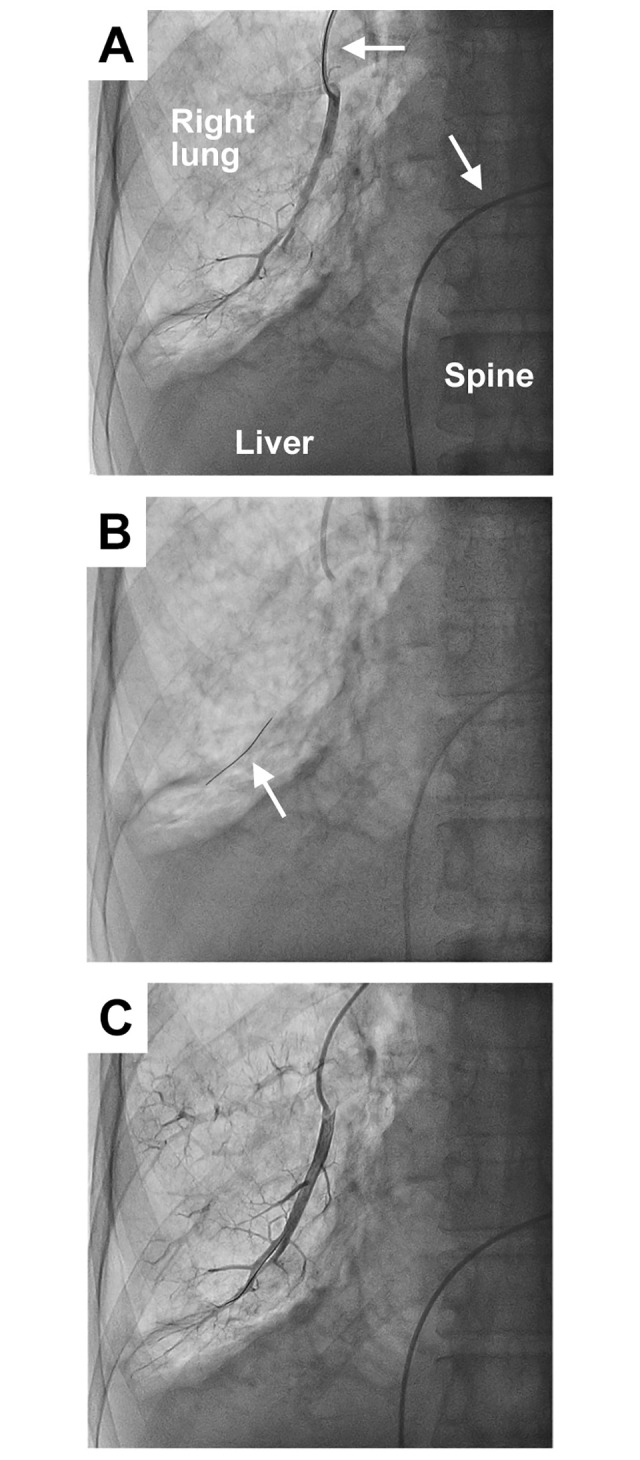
Representative X-ray frontal images during cardiac catheterization. (A) The pulmonary arteries in the lower lobe of the right lung are shown using contrast medium. The suitable pulmonary artery to facilitate the placement of a temperature- and pressure-sensor guidewire was selected with an angiogram. The white arrows indicate a guiding catheter used to inject the contrast medium and deliver the guidewire. The catheter was delivered from the inferior vena cava to the right central pulmonary artery. (B) The white arrow indicates the guidewire placed distally to the pulmonary artery to measure blood pressure and mean transit times in the artery. (C) A final angiogram following the above measurements indicated no occurrence of vascular complications in the artery.
